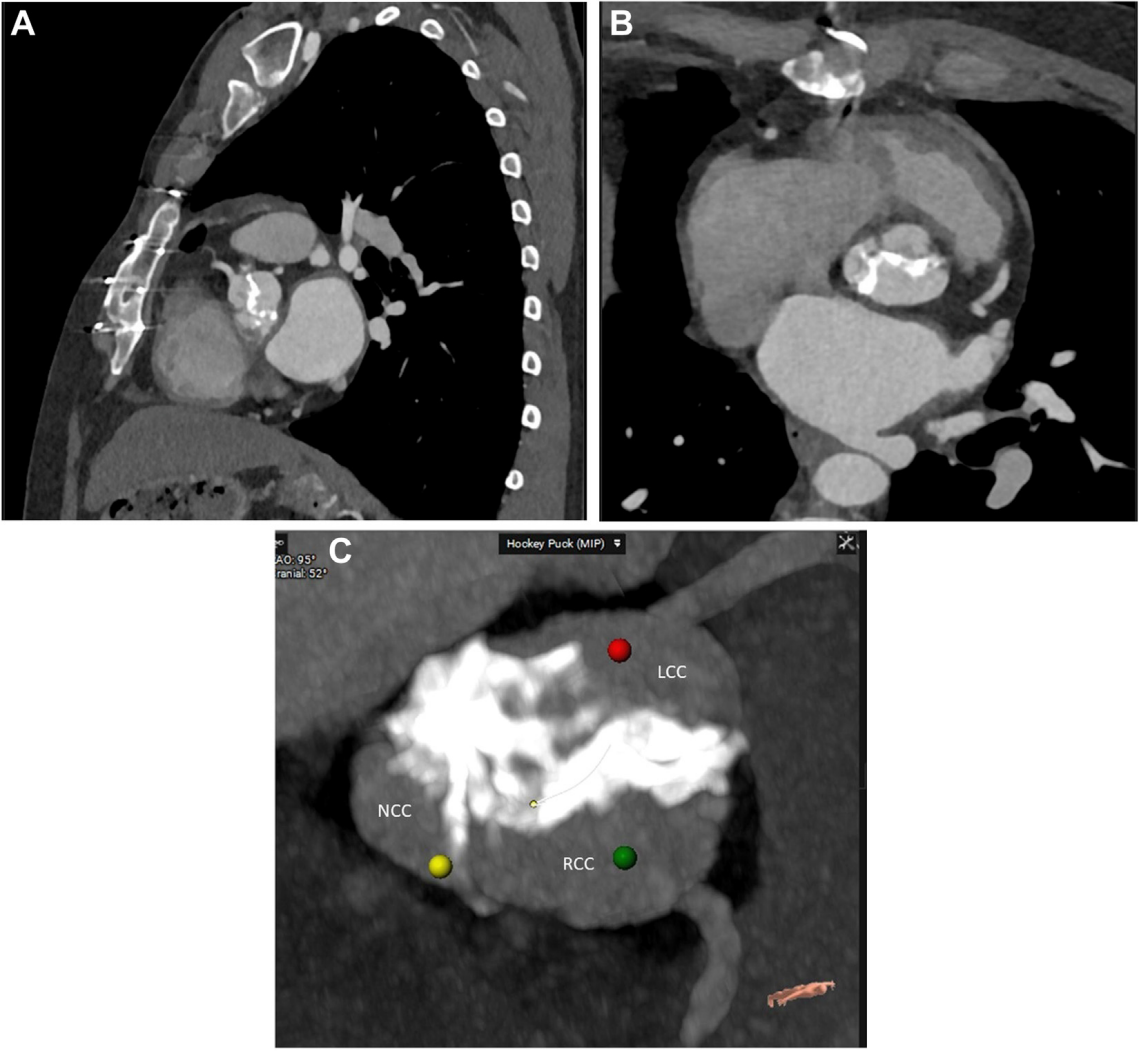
Computed Tomography Imaging of the Degenerated AVNeo Sagittal (A) and axial (B, C) computed tomography scan showing calcification and thickening of the left neo-valve leaflet. LCC = left coronary cusp; NCC = noncoronary cusp; RCC = right coronary cusp.
